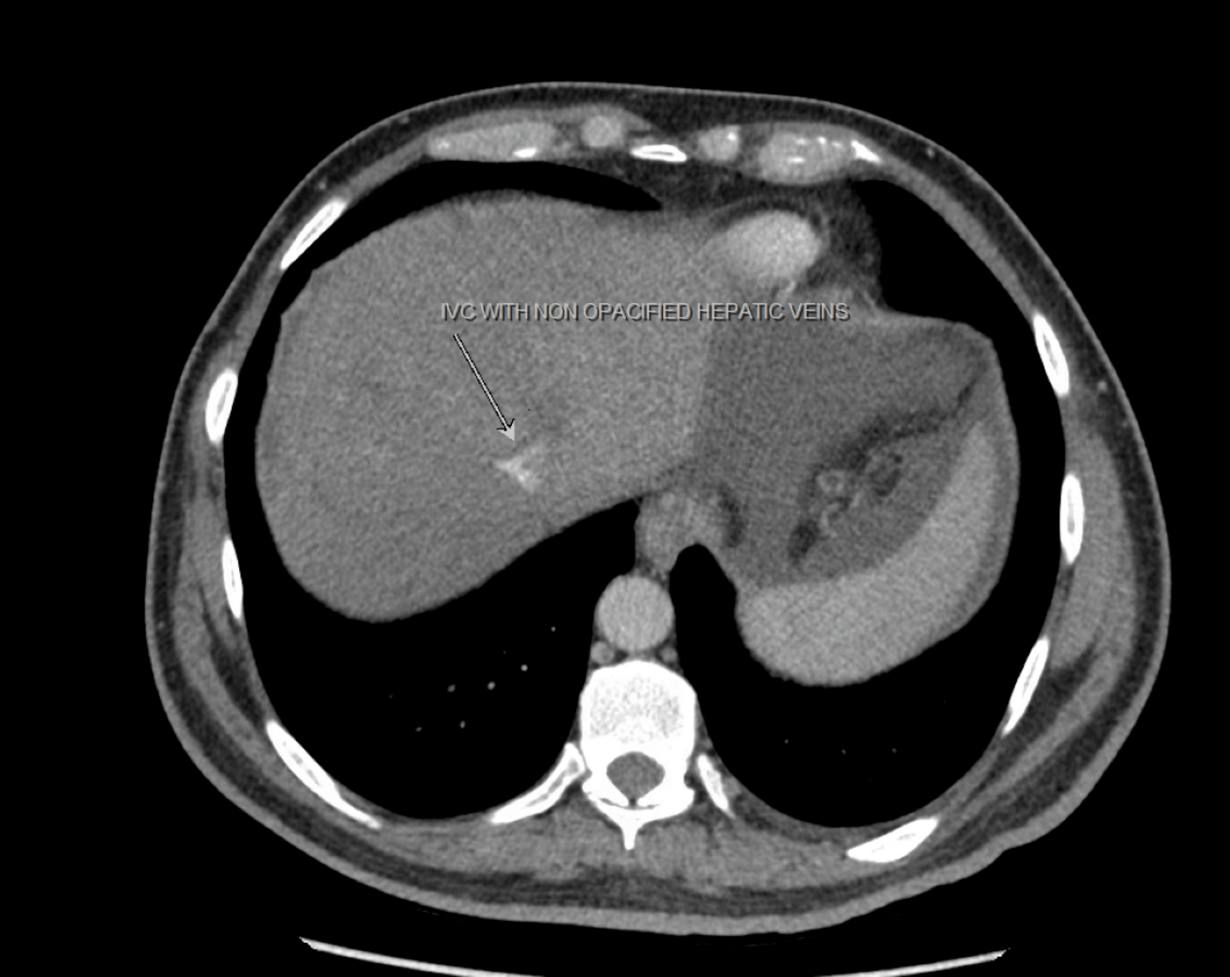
Abdominal CT with contrast showed the attenuation of hepatic veins (marked by the white arrow)
